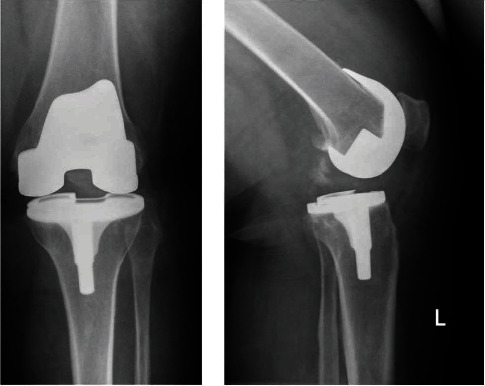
(a) Anteroposterior radiograph of case 4 at presentation in the emergency room. (b) Lateral radiograph of case 4 at presentation in the emergency room.
